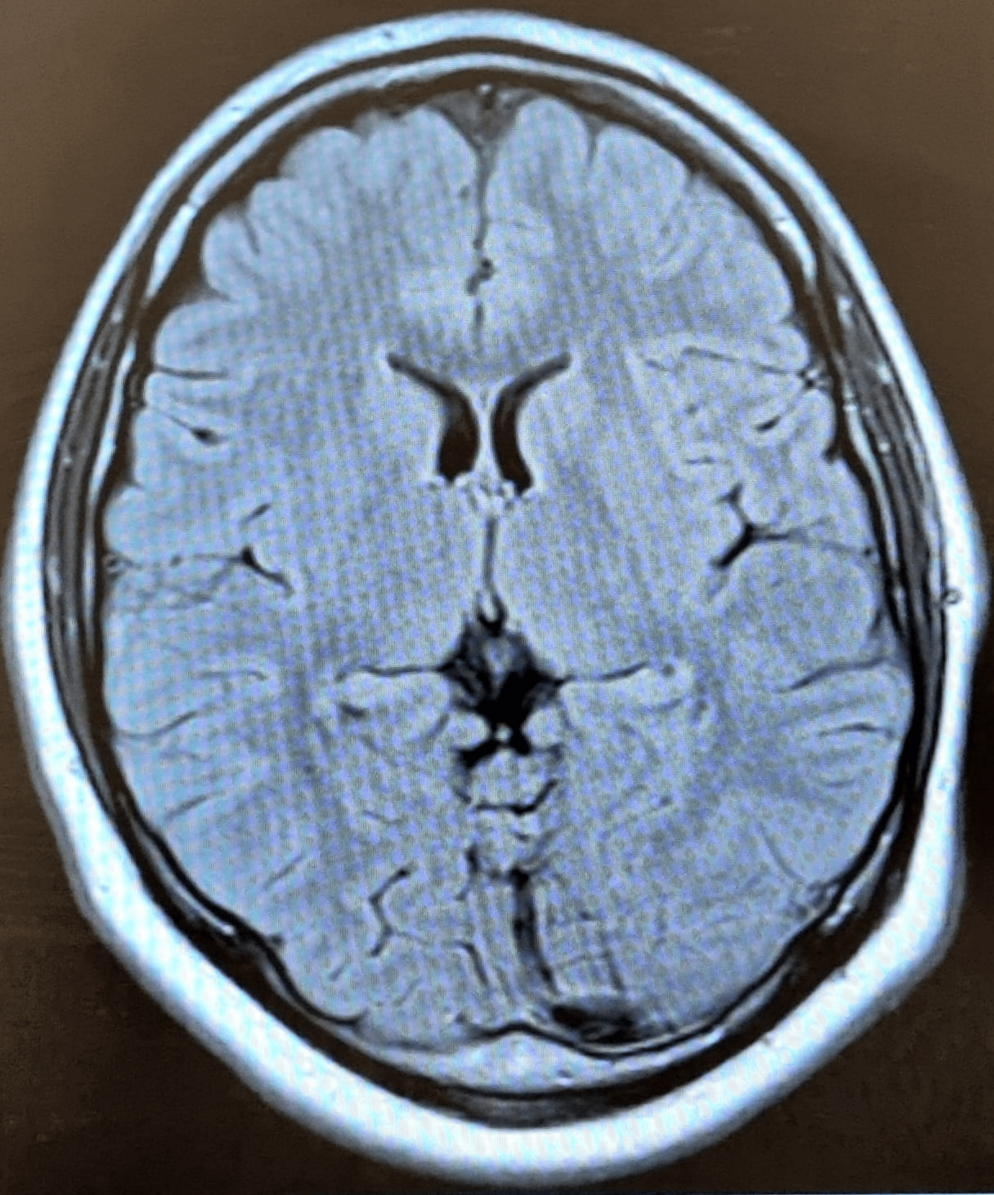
The MRI of Case 1's brain (axial FLAIR image) shows no gross pathology. FLAIR: fluid-attenuated inversion recovery
